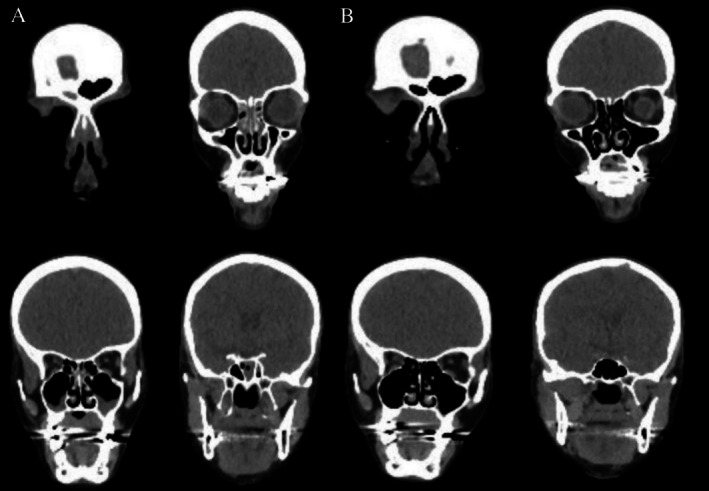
(A–B): Patient 1 CT Imaging of sinuses before (A) and after (B) completing 2 months of steroid therapy for immune‐mediated sinusitis.
